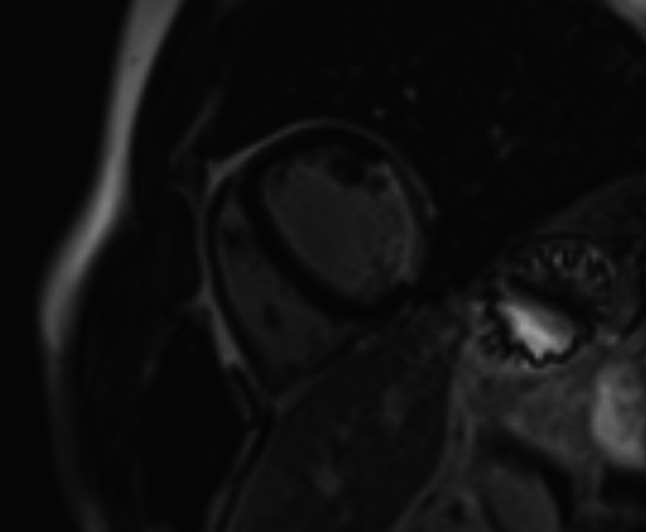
Cardiovascular MRI with gadolinium contrast showing transmural myocardial infarction of inferolateral wall
